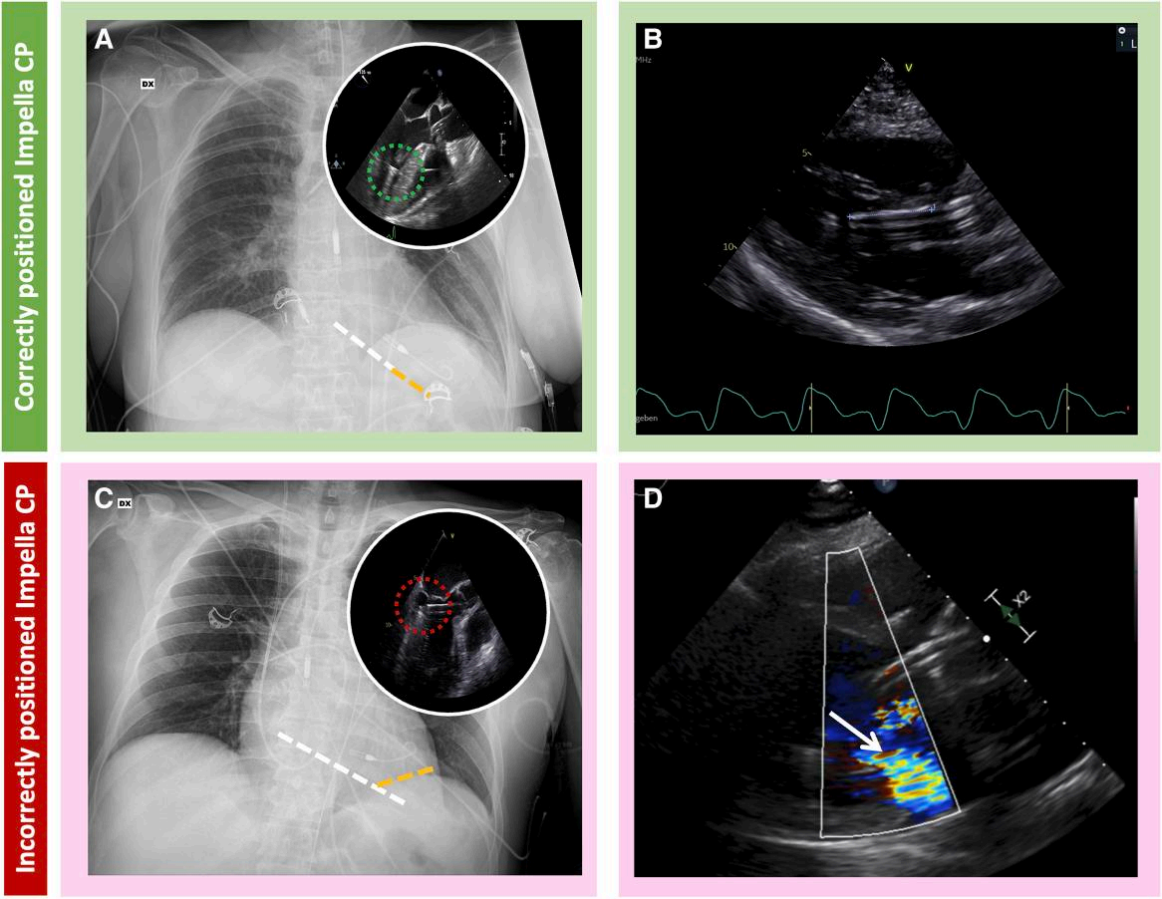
Echocardiography in Impella CP. (*A*) Correctly positioned Impella CP with chest radiography showing the major axis of the distal Impella shaft (white dashed line) and the pigtail axis (yellow dashed line); therefore, the pigtail bending angle is 0. TOE in the same panel is showing mid-oesophageal long-axis view at 120° with the Impella inlet (green dashed circle) in the apex. (*B*) Left parasternal long-axis view showing a correctly positioned Impella CP with the inlet located 4 cm from the aortic valve annulus. (*C*) Malrotated Impella CP with chest radiography showing the major axis of the distal Impella shaft (white dashed line) and the pigtail axis (yellow dashed line); therefore, the pigtail bending angle is 45°. TOE in the same panel is showing mid-oesophageal long-axis view at 120° with the Impella inlet impinging on the LV inferolateral wall (red dashed circle). This example demonstrates a crushed pigtail sign. (*D*) Left parasternal long-axis view showing an incorrectly positioned Impella CP with the device impinging on the anterior mitral leaflet leading to MR (white arrow).
